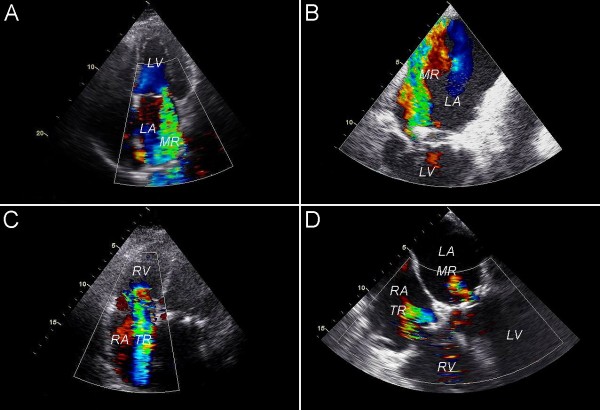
Doppler echocardiographic imaging.A. Preoperative TTE; grade 4+ MR. B. Postoperative TEE; grade 4+ recurrent MR along the posterior annulus. C. Postoperative TTE; grade 4+ TR. D. Two-year follow-up TEE; grade 1+ residual TR and MR. LA, left atrium; LV, left ventricle; MR, mitral regurgitation; RA, right atrium; RV, right ventricle; TEE, transesophageal echocardiography; TR, tricuspid regurgitation; TTE, transthoracic echocardiography.
